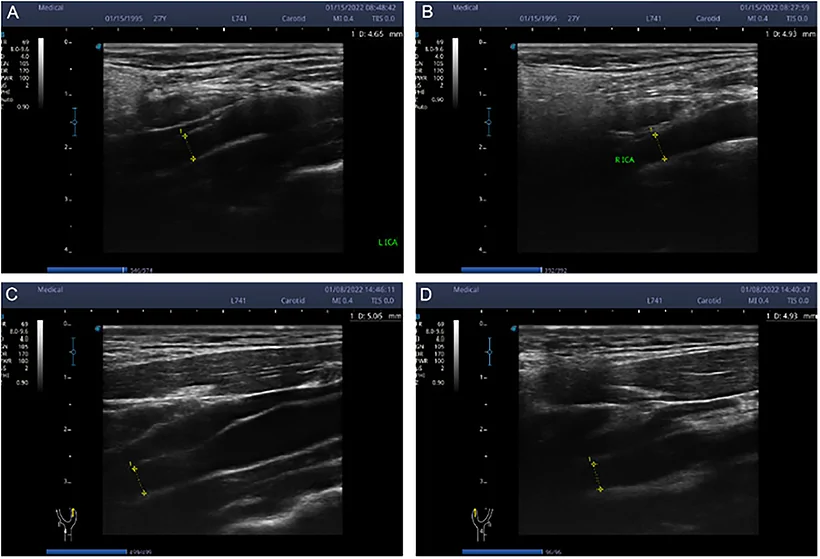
The ultrasonic images of two participants to show diameter measurement for the internal carotid artery (ICA) using carotid ultrasonography. (A‐B) the diameters of the left ICA and right ICA of participant 1; (C‐D) the diameters of the left ICA and right ICA of participant 2.
